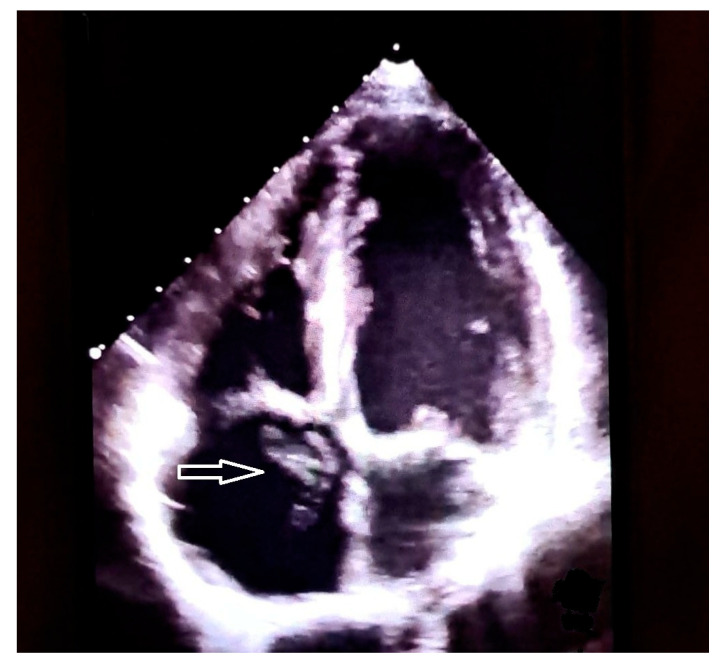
Transthoracic echocardiogram (4 chamber-view) showing a thin and mobile membrane floating in the right atrium (Chiari’s network, white arrow).
